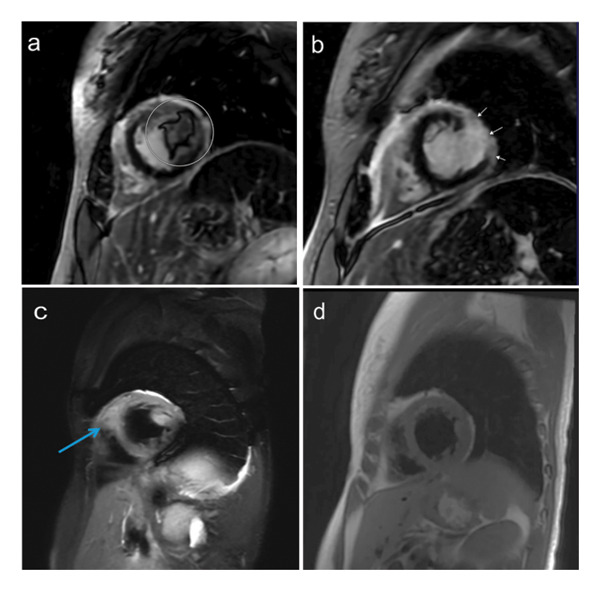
Cardiac delayed enhancement MRI imaging pretreatment (a and c) and 2.5 months posttreatment with anticoagulation and complement inhibition (b and d). LV thrombus seen in (a) (circle), with replacement of thrombus by mildly enhancing scar tissue laterally (arrows) in (b). RV thrombus seen in (c) with near‐resolution after 2.5 months of treatment (d).
